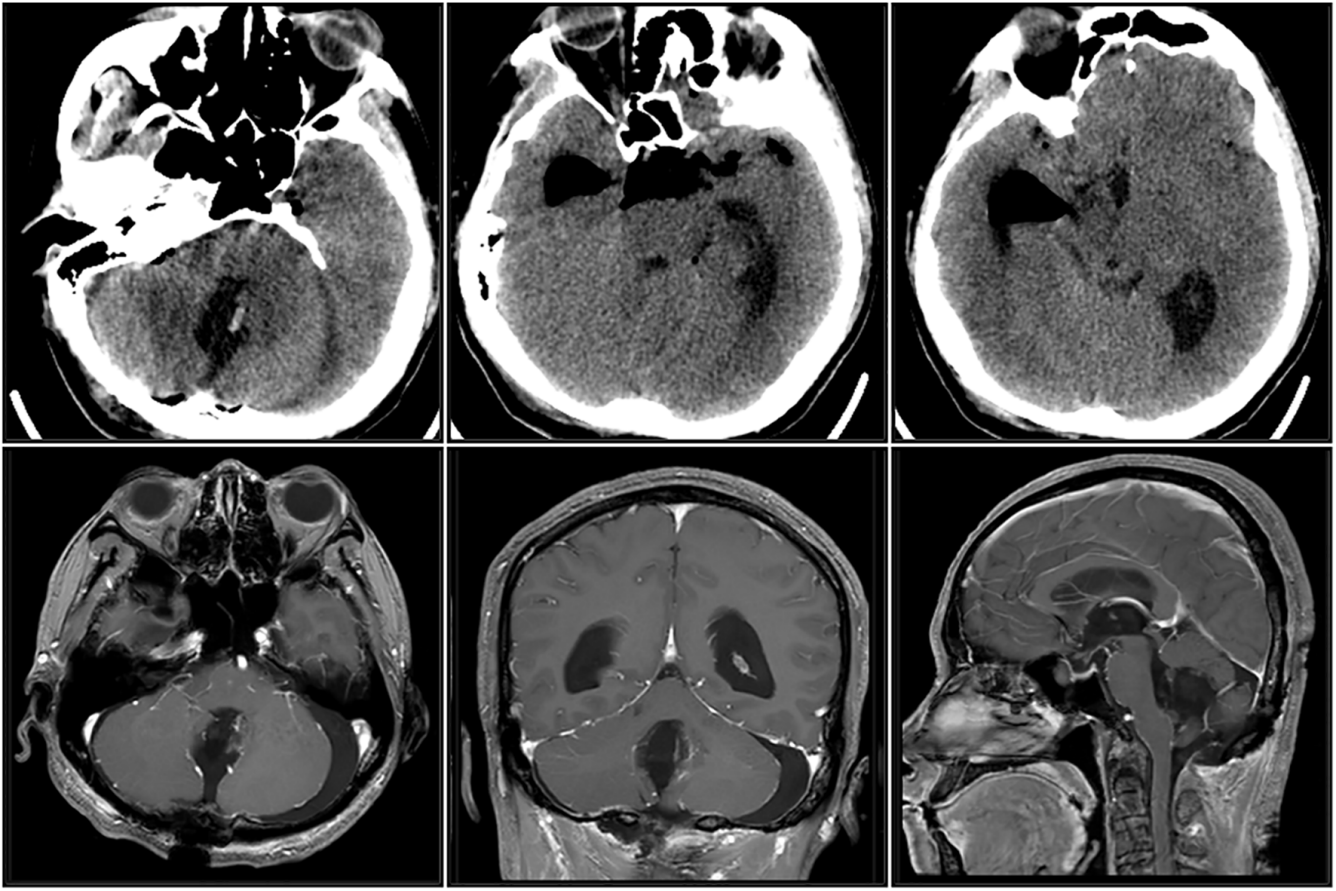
Postoperative CT and MRI.
